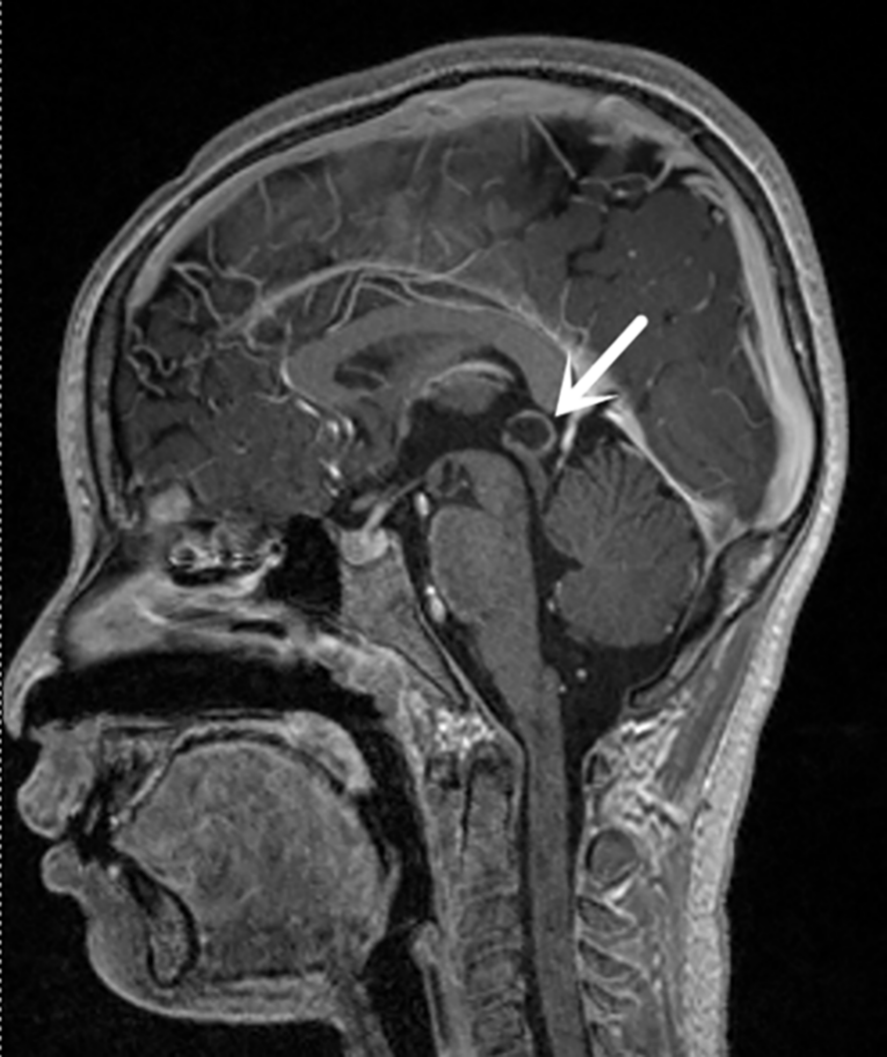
Sagittal post-contrast T1-weighted MRI demonstrating a well-defined rounded cystic lesion in the pineal region extending into the quadrigeminal cistern, consistent with a pineal cyst. White arrows indicate the pineal cyst location in sagittal MRI image.
